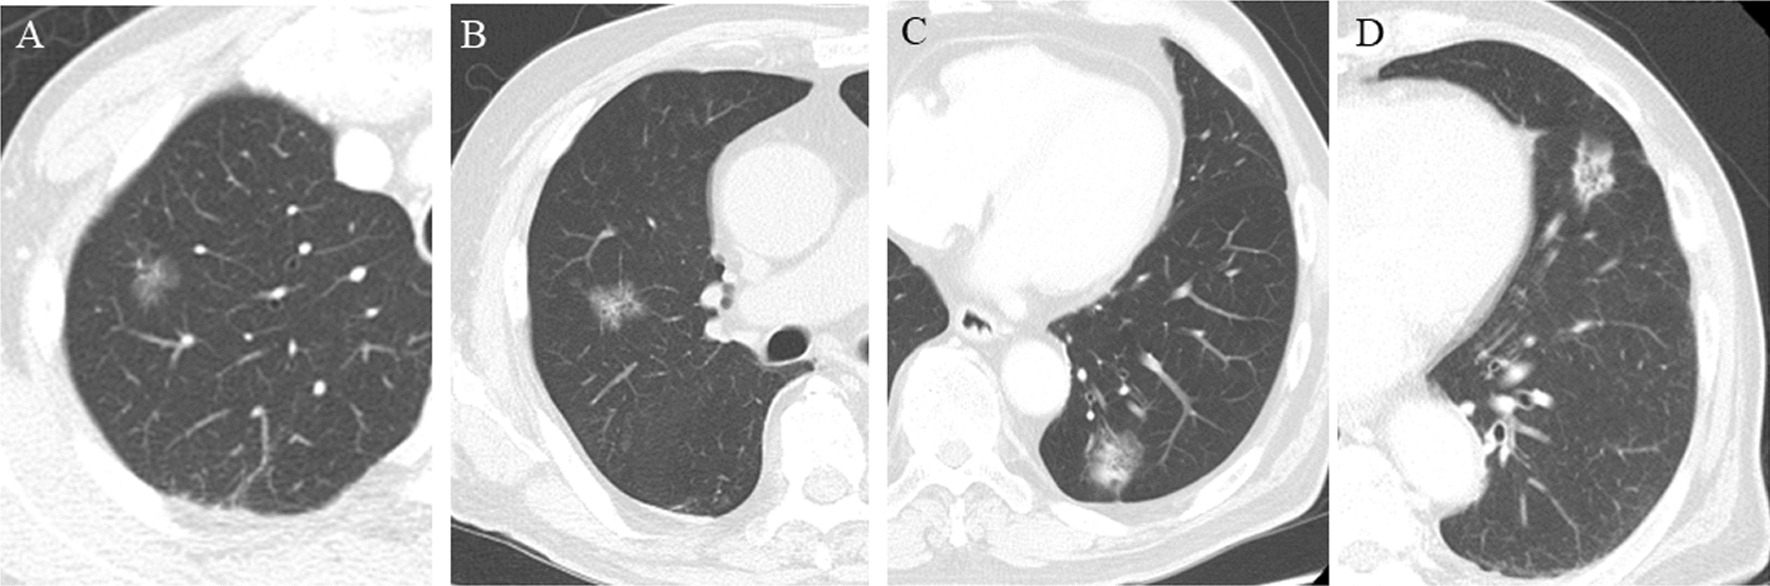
Comparison of the mean computed tomography (m-CT) values. The m-CT value was A − 701.8, B − 530.4, C − 462.5, D − 295.1 Housfield units (HU)
